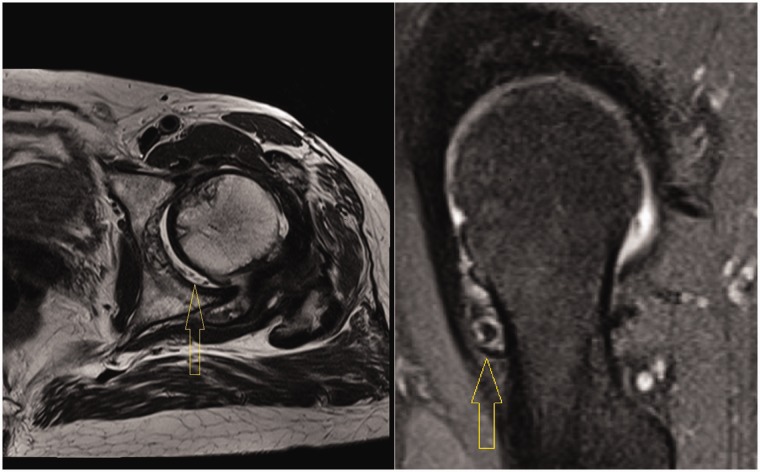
MRI showing loose bodies in the joint space, in two different cases.
